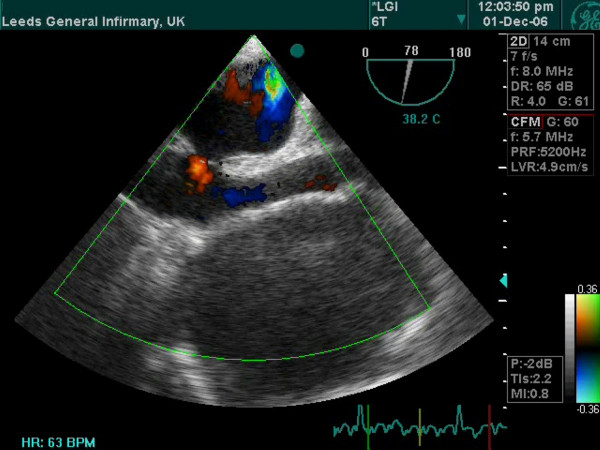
Transesophageal echocardiogram shows a huge anterior mediastinal fluid filled mass.
